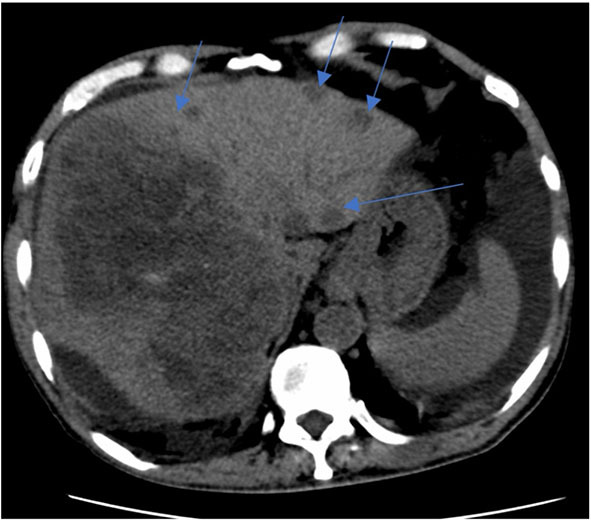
CT liver 4 phase. Key images of two scans of the same patient, at the same slice taken 3 weeks apart. Subsequent CT shows enlarging right liver mass with multiple new left liver lobe lesions (multiple blue arrows).
